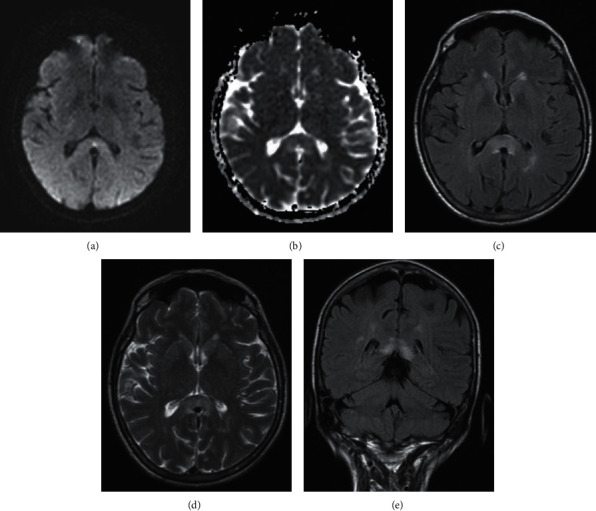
MR brain with and without contrast on admission. (a) DWI, axial cut. (b) ADC axial cut. (c) FLAIR axial cut. (d) T2 axial cut. (e) FLAIR coronal cut. Imaging reveals hyperintensity in the splenium of the corpus callosum.
